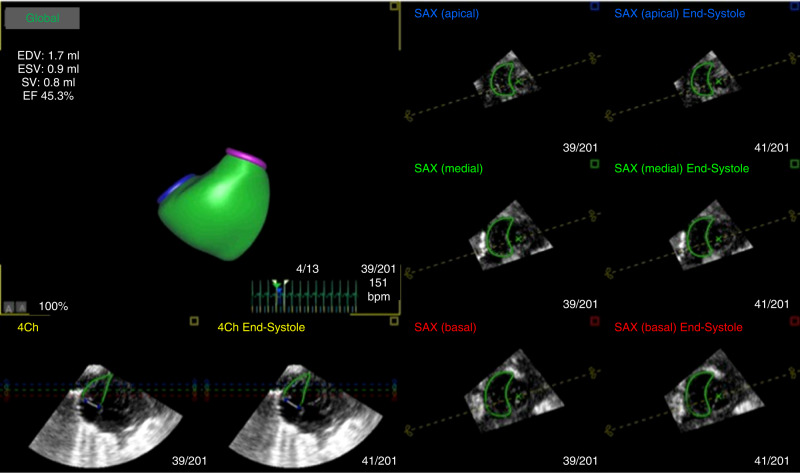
Offline analysis of three-dimensional echocardiographic right ventricular (RV) volume calculations at end-diastole. Green lines indicate semi-automatically detected RV internal border. EDV end-diastolic volume, ESV end-systolic volume, SV stroke volume, EF ejection fraction, SAX short axis, 4Ch four chamber.
